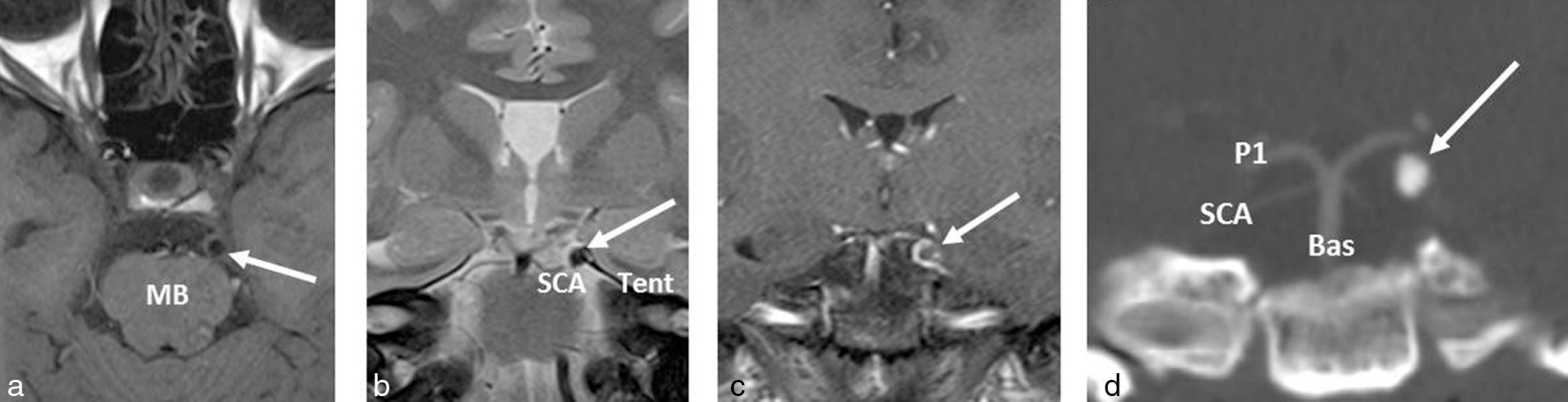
Axial *T*_1_ (a) and coronal *T*_2_ weighted fat-saturated (b) MRI showing a well-defined, small, rounded, hypointense lesion (arrows) in close relationship to the left tentorium (Tent) and left SCA at the level of the MB. The lesion (arrow) demonstrates thin peripheral enhancement on coronal *T*_1_ weighted fat-saturated post-contrast imaging (c). On coronal maximum intensity projection reconstructions of CT angiogram of circle of Willis (d), the lesion (arrow) demonstrates density consistent with calcification and lies in the expected location of the proximal left cisternal third nerve, between the SCA and P1 segment of the left posterior cerebral artery, with no vascular connection, which was also confirmed on catheter angiogram (images not shown). Bas, basilar artery; MB, midbrain; SCA, superior cerebellar artery.
